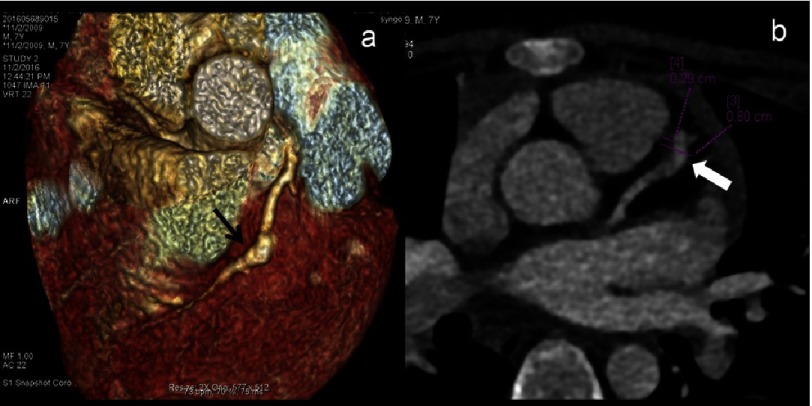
(a–b): CTCA VRT (a) axial (b) images of a 7 year male child in convalescent phase (4 years after diagnosis) show fusiform aneurysmal dilation (a - thin arrows) of proximal LAD; (b) shows a giant aneurysm with eccentric thrombus along the anterior wall (thick arrow). 2D-transthoracic echocardiography though could detect aneurysm but thrombus was missed.
